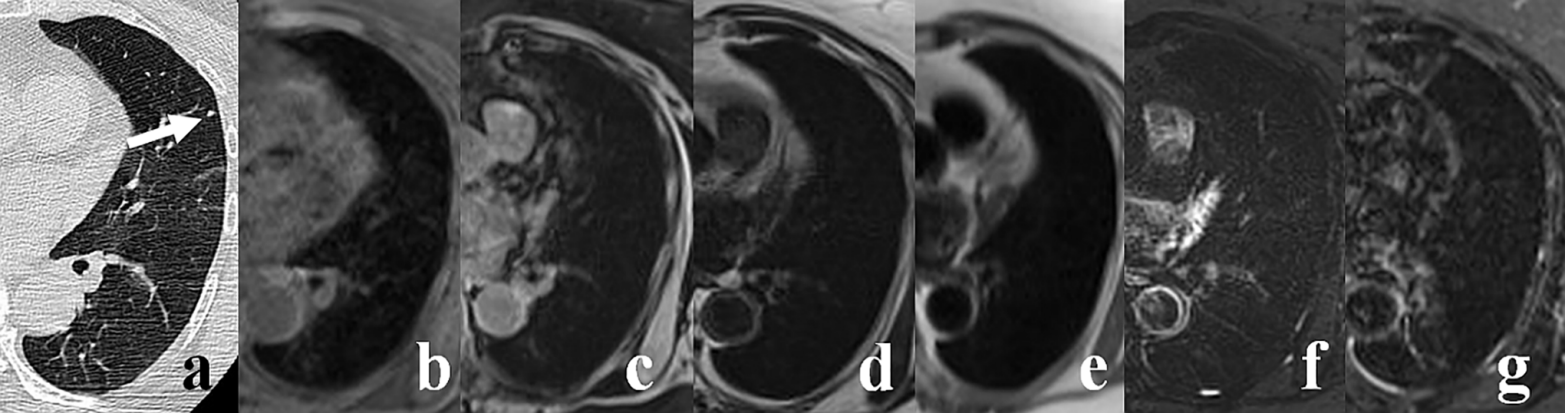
A 77-year-old woman with 2.8 mm pulmonary nodule (arrow) in the left upper lobe (lingula). The nodule is well depicted on MDCT image (A), however it was not identified on corresponding MR images: T1 VIBE (B), T1 out-of-phase (C), T2 TSE (D), T2 HASTE (E), T2 SPIR (F), T2 STIR (G).
